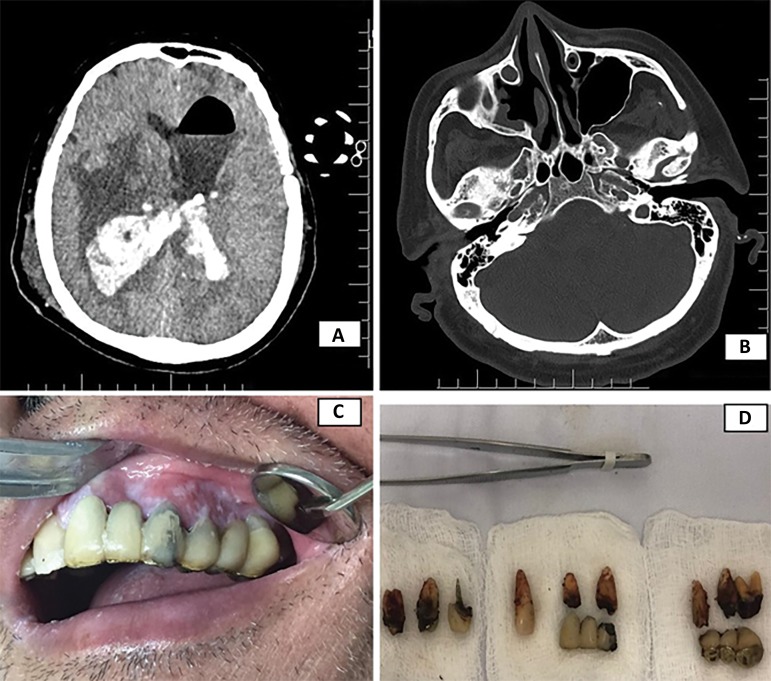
Clinical and imaging aspects of the patient with infection. (A) Computed tomography image showing a pre-existing brain injury. (B) Encapsulated regular mass and hyperdense area filling a portion of the maxillary sinus space on the right side. (C) Initial clinical appearance showing a poor state of preservation and foci of infection. (D) Dental elements after extraction.
